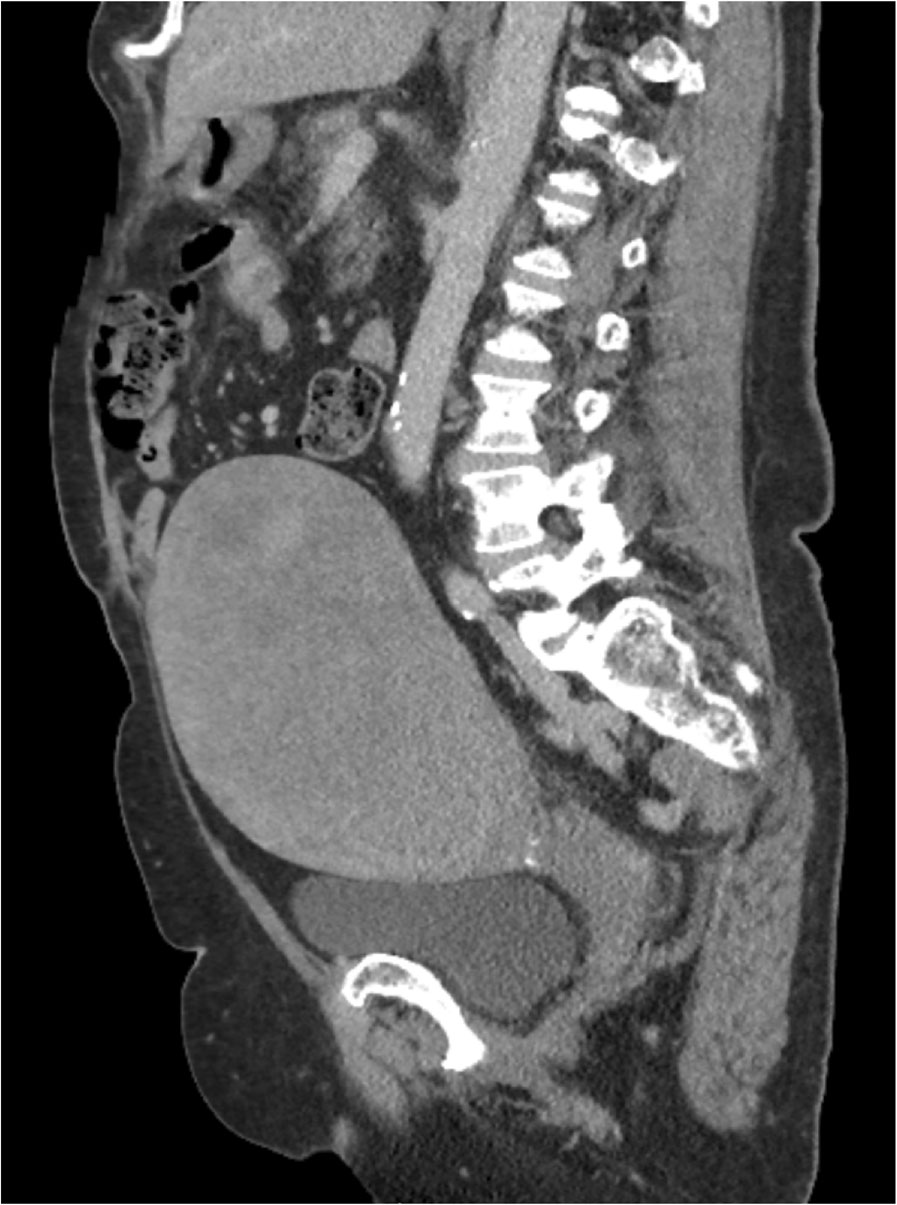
CT pelvis showing the large necrotic uterine mass
